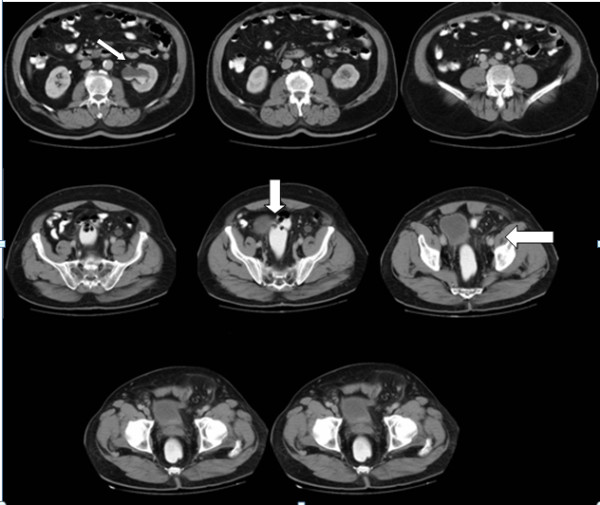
Preoperative abdominopelvic computed tomography scan. Left hydroureteronephrosis (thin arrow). Distal ureteral obstruction and bladder displaced by entrapped herniated sigmoid colon (thick arrow).
